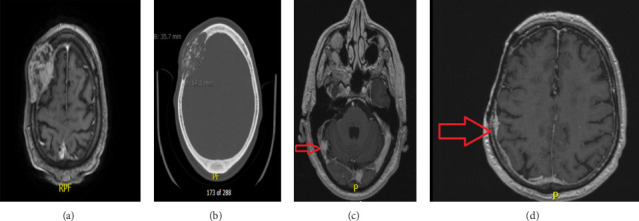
(a, b) Preoperative MRI and CT scan showing the right frontal skull osteosarcoma with characteristic expansion and sunburst appearance with evident cortical destruction. (c) MRI axial images T1 with contrast showing right transverse sinus lesion with heterogeneous intensity and dense contrast uptake. (d) MRI T1 with contrast showing thickened enhanced right-sided dura as evidence for distant metastasis.
